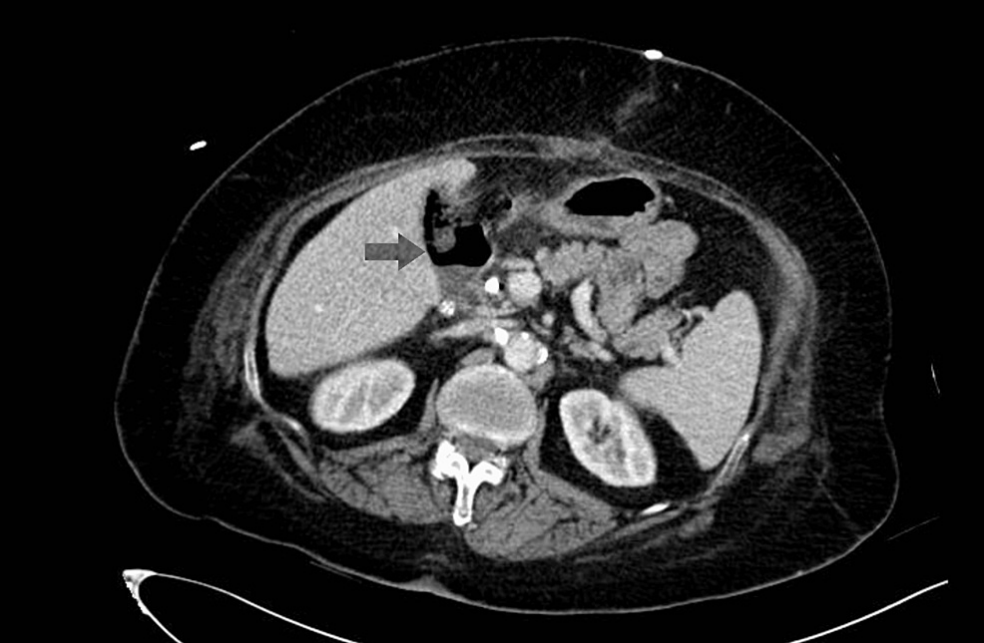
CT abdomen showing the sub-hepatic collection with foci of gas
